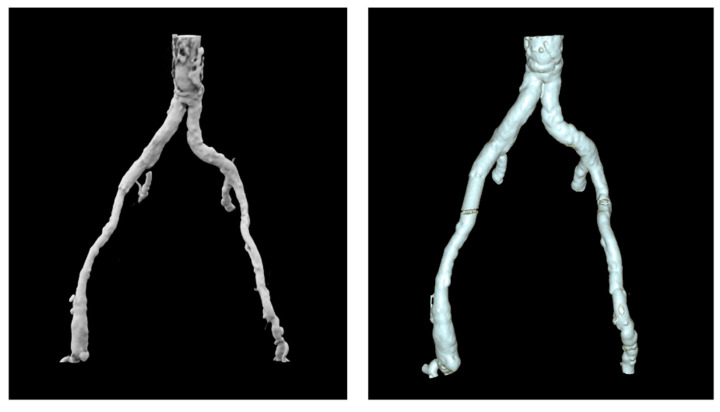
Comparison between the 3DVR of a patient’s CT scan (left) and the 3DVR of the respective 3D-printed model’s CT scan (right).
